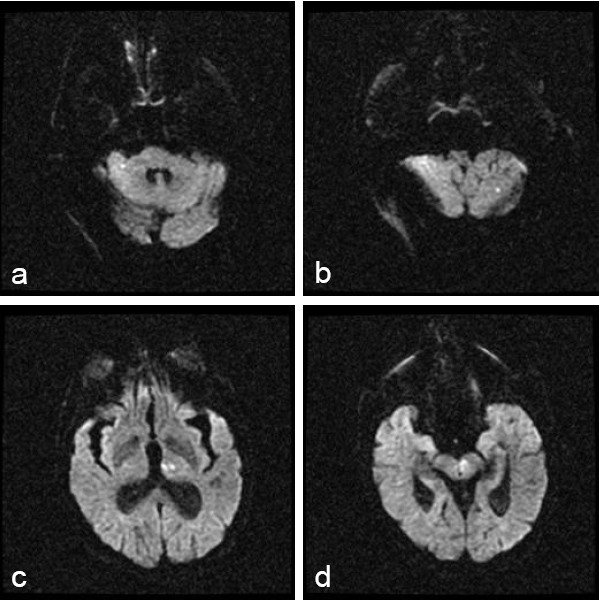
Diffusion-weighted magnetic resonance image showing punctate diffusion bright lesions in the cerebellum (panels a and b), thalamus (c), and midbrain (d).
